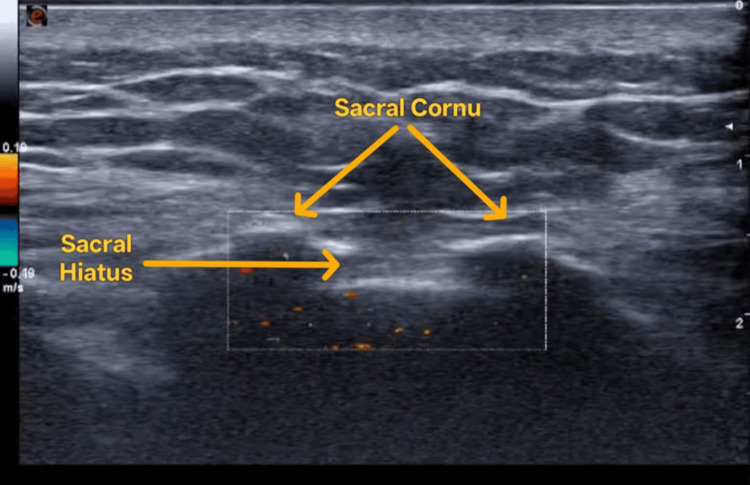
Ultrasound image showing transverse view of the sacral hiatus
